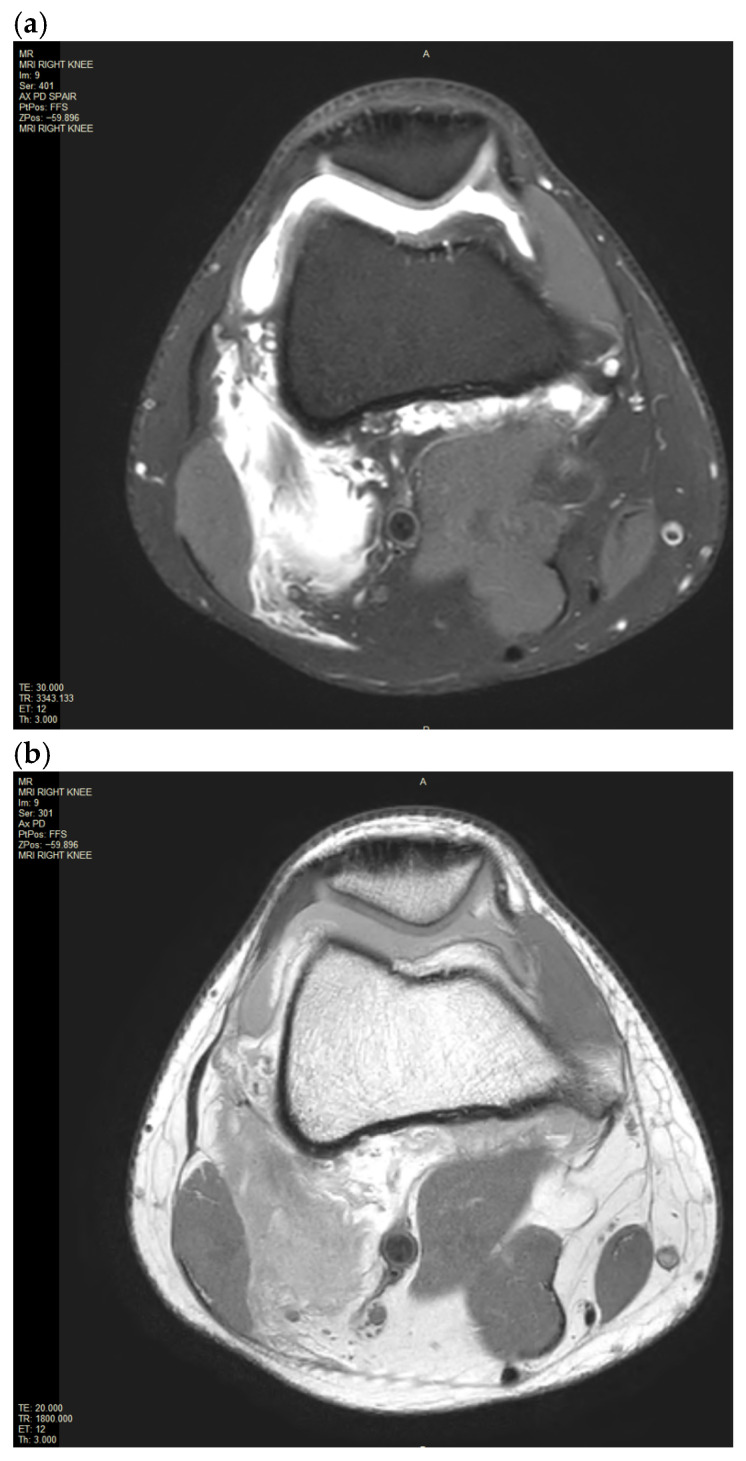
(a,b). MRI of the right knee image of the moderate knee joint effusion into the supra-patellar recess associated with incomplete avulsion of the lateral gastrocnemius origin as well as intramuscular haemataoma and partial avulsion of the medial gastrocnemius origin on Coronal PD PD SPAIR and PD TSE.
